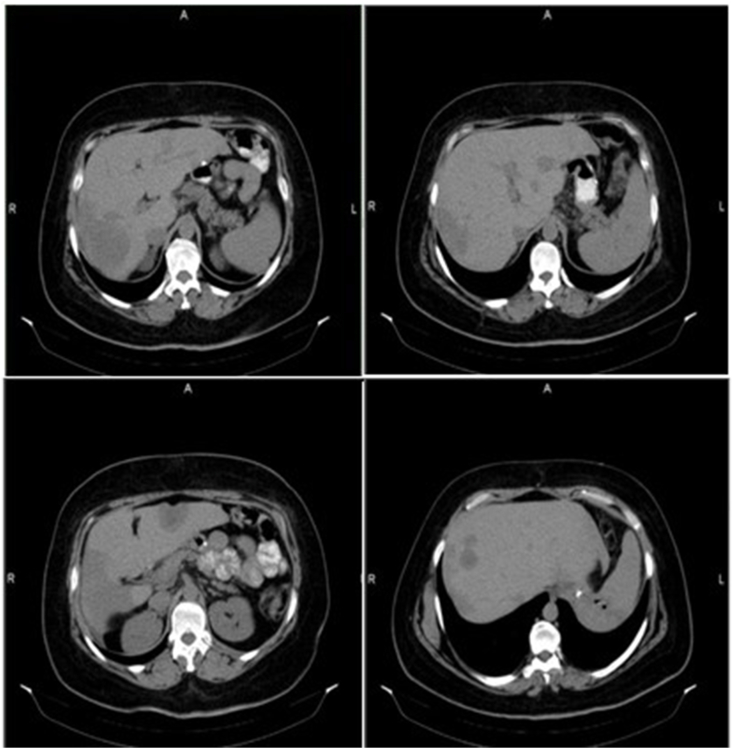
A computed tomography scan at the beginning of diagnosis of a patient with primary hepatic epithelioid hemangioendothelioma. The scan revealed a triangular hypodense lesion in the periphery of the seventh hepatic segment. Multiple other hepatic lesions were also observed, with the largest measuring 4 cm in the anterior segment of the fourth hepatic segment. In addition, there was a right adrenal mass measuring 2.5 cm, which was consistent with a benign adenoma.
